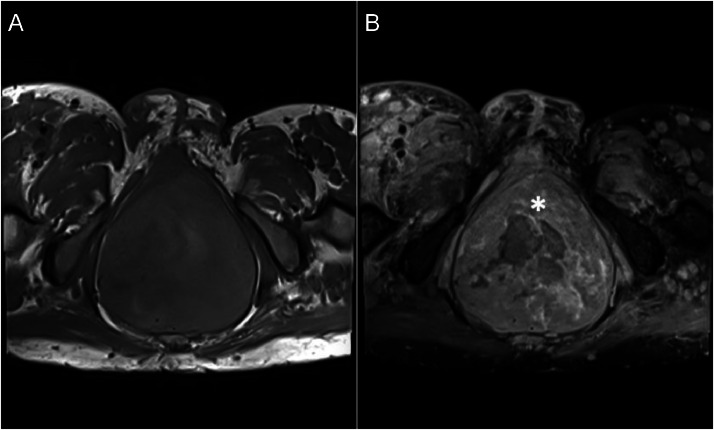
MRI axial projection showed noncontrast T1-weighted imaging (A) showed a giant mass with solid and necrotic area arising from the prostate, measuring approximately 9.2 × 19.3 × 9.6 cm, extending to the abdominal cavity, which, on gadolinium contrast-enhanced T1-weighted fat-suppressed imaging (B) exhibited heterogenous contrast enhancement on solid components (asterisk).
